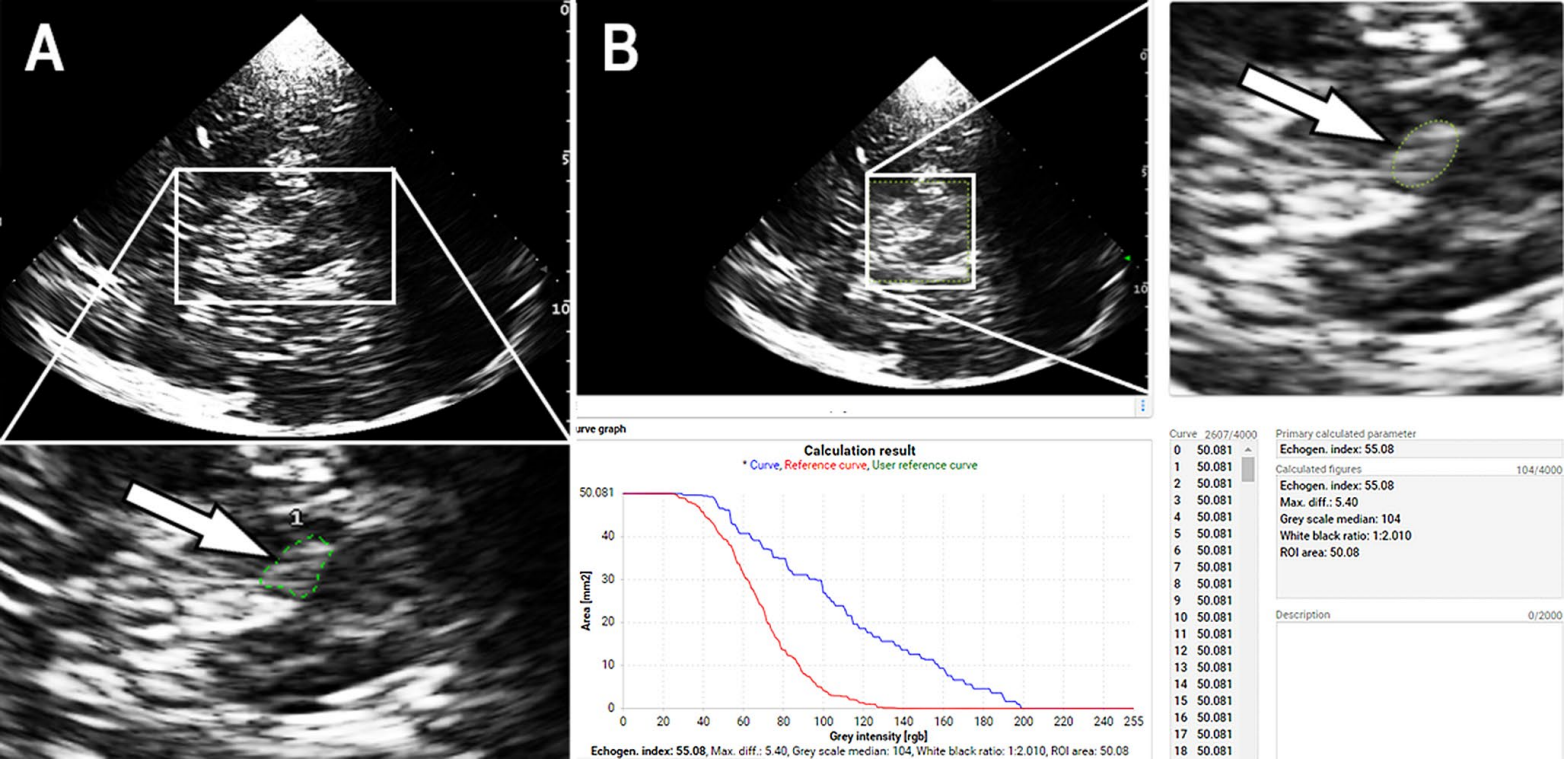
Visualization and measurement of substantia nigra echosignals in Parkinson’s patients. (A) Transcranial brain sonogram of axial transection at caudal midbrain level. The lower panel shows the zoomed midbrain in which the substantia nigra echo signals (arrow) on the side ipsilateral to the insonation were manually surrounded for planimetric measurement of echogenic area. (B) Screen shot of the MATLAB-based software tool “Cereb B-Mode Assist” used for digitized quantification of substantia nigra echo-intensity. The right panel shows the zoomed midbrain in which an elliptical ROI of 50 mm^2^ was placed to cover the echo signals of the substantia nigra (arrow) on the side ipsilateral to the insonation. The automatic analysis results in the curve of the echo-intensity distribution in the ROI compared to a reference curve determined in healthy controls (lower left panel) and the calculated index value of the total substantia nigra echo-intensity (lower right panel)
